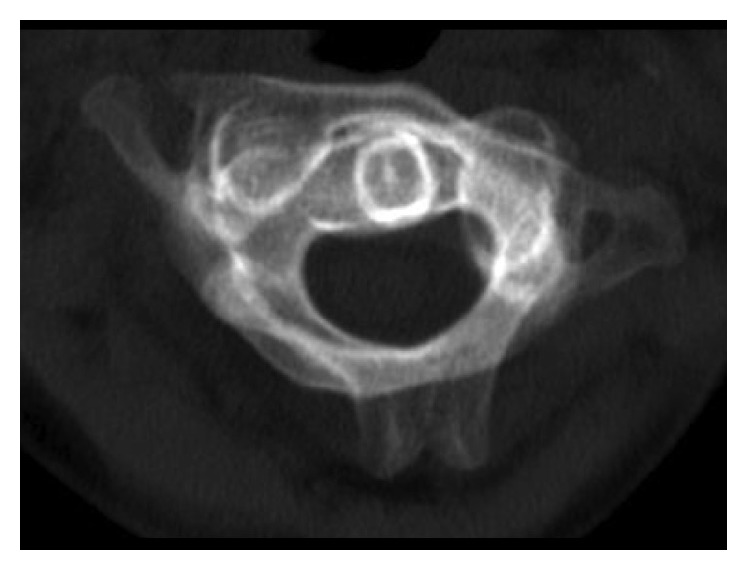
The initial axial CT scan of C1-C2 demonstrates rotatory displacement, CT-MIP (maximum intensity projection).
